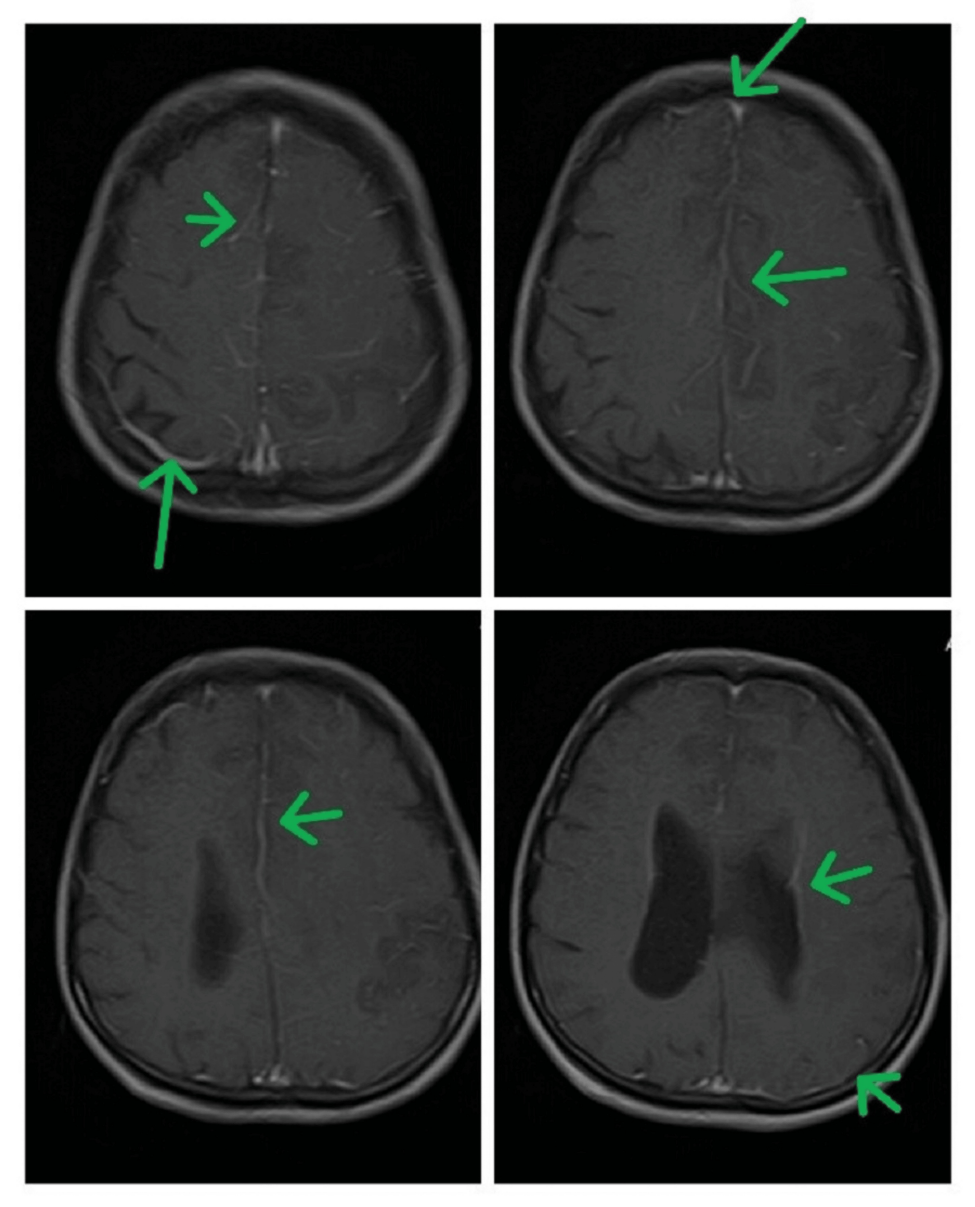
T1-weighted imaging (T1WI) MRI post-contrast images show leptomeningeal enhancement (green arrows)
